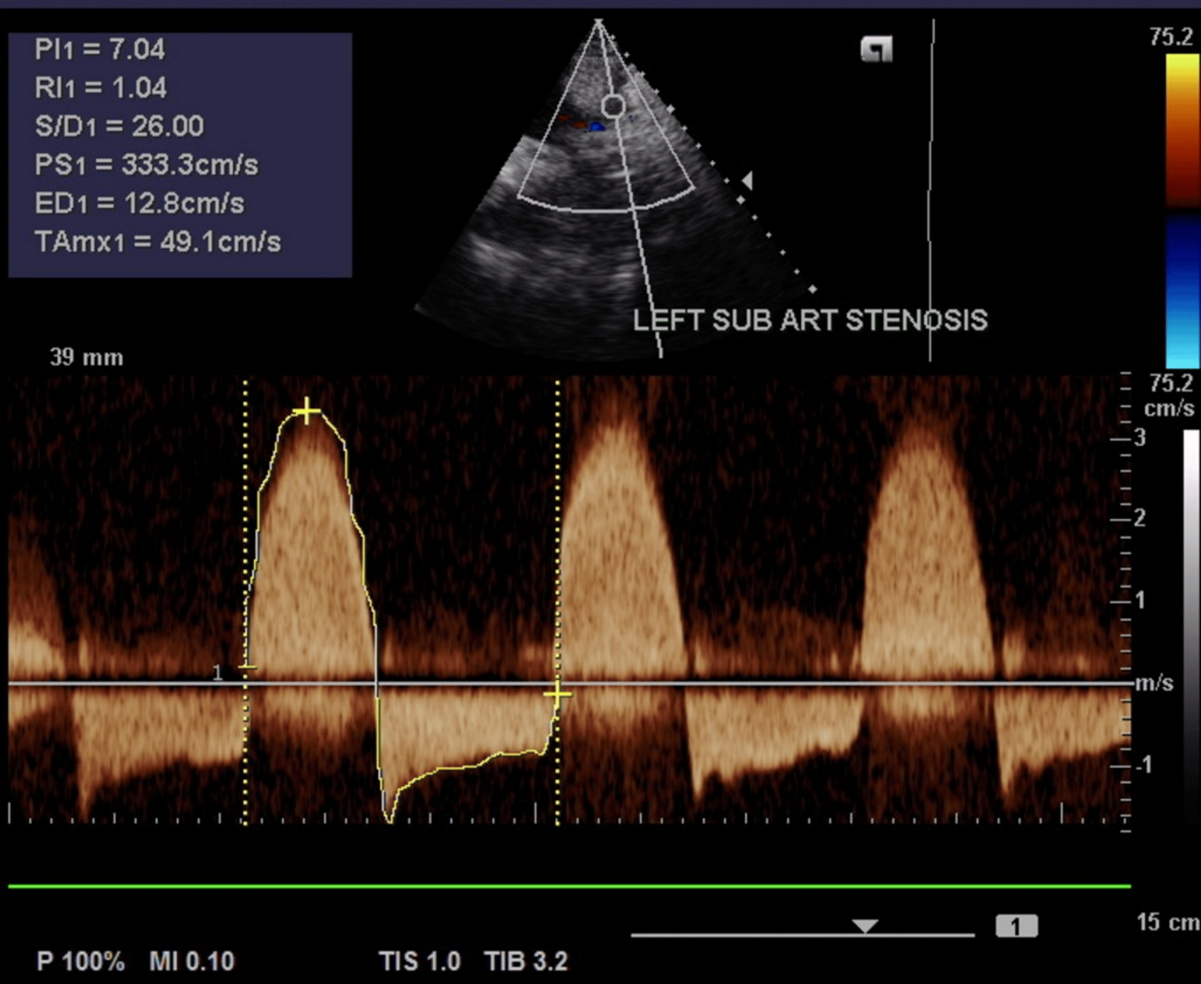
Spectral Doppler (continuous wave Doppler) of the proximal segment of the left subclavian artery. Note the high peak velocities of 333 cm/s (normal range 45-180 cm/s) P: positivity; R: resistivity; S/D: systolic/diastolic; PS: peak velocity; ED: end diastolic velocity; TAmax: mean volocity
